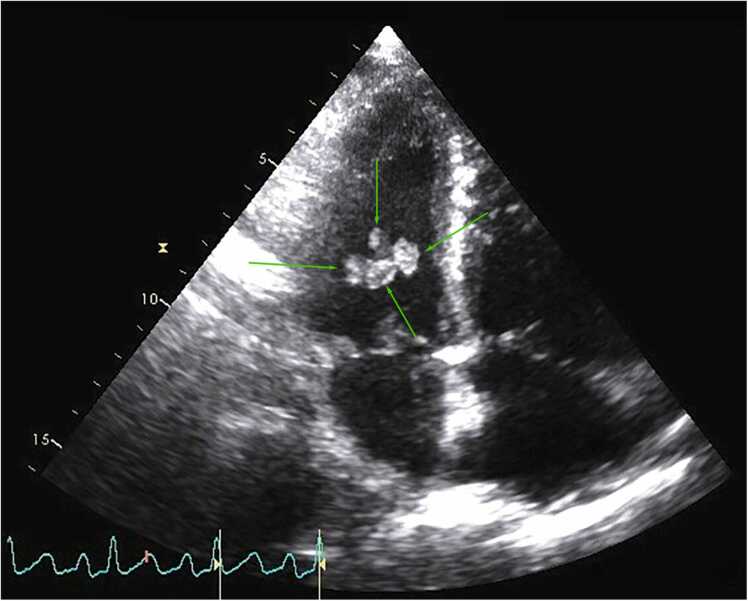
Apical four chamber view with arrows pointing to a four ovoid masses on the mitral chordae. Video clip available.
